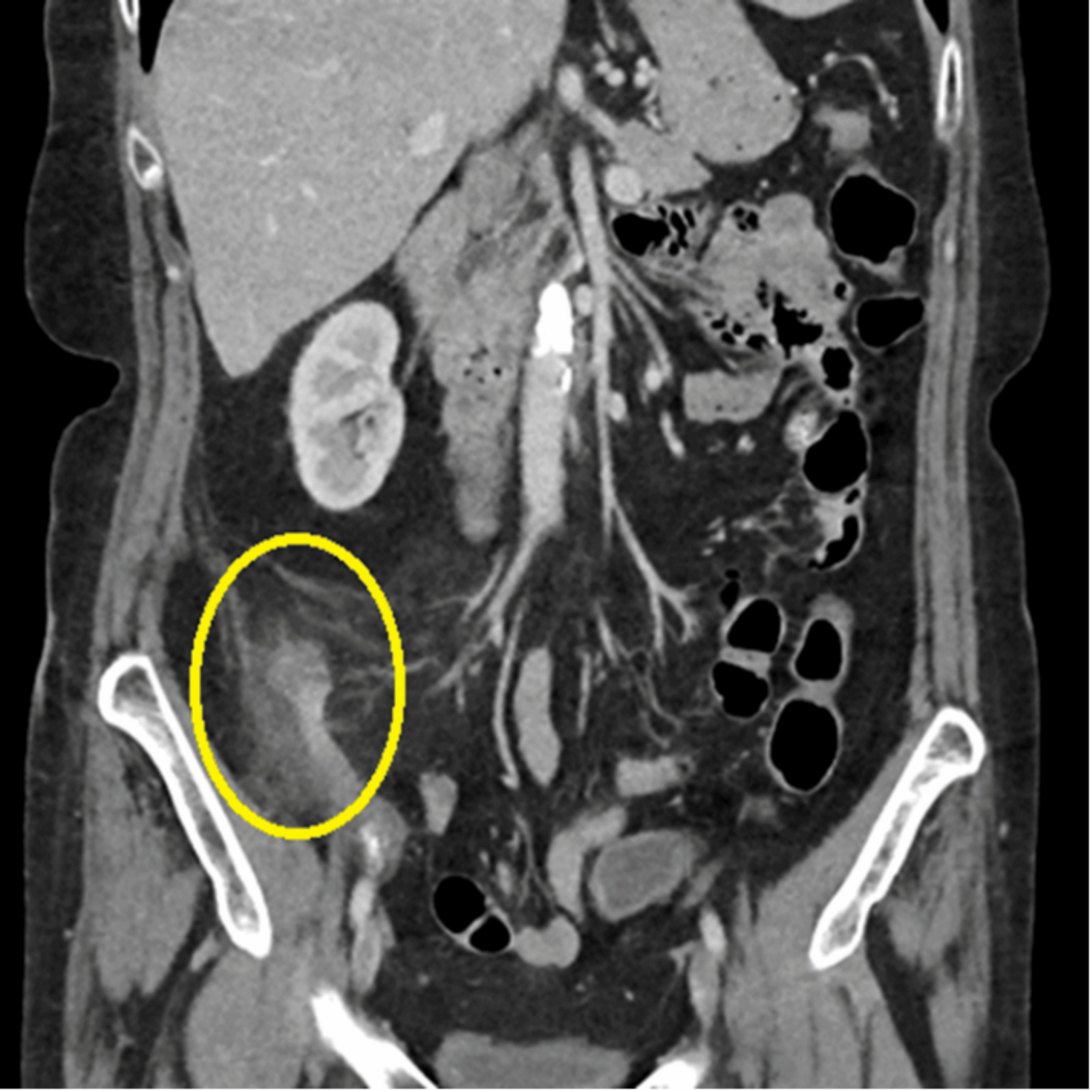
Coronal CT with contrast imaging of the abdomen Circled in yellow is the thickened appendix with extensive inflammatory changes surrounding the appendix, especially evident in the distal tip.
